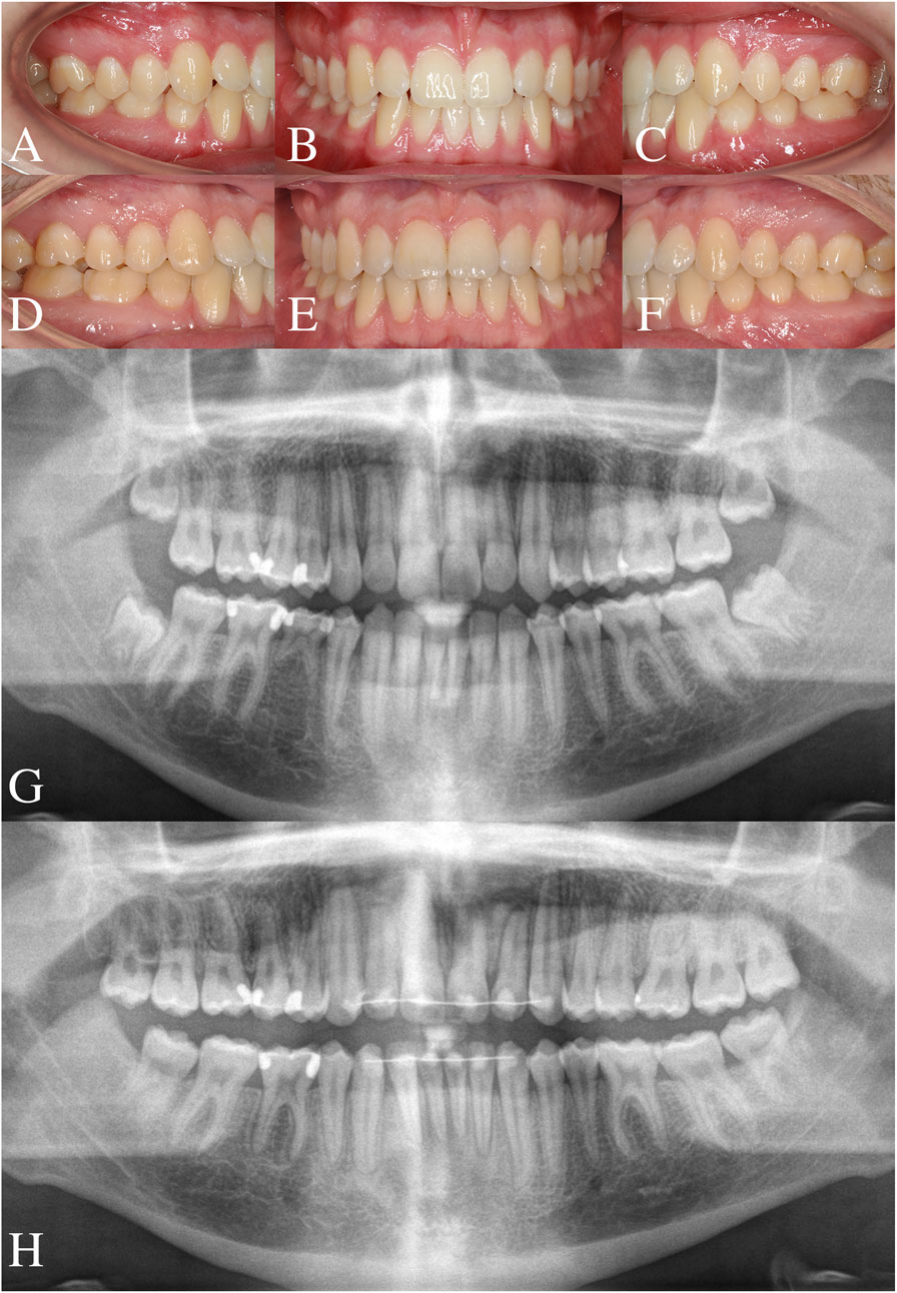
A typical case of unilateral space closure. a–c, Pretreatment photographs. d–f Post-treatment photographs. g, h Pre- and post-treatment panoramic radiograph
